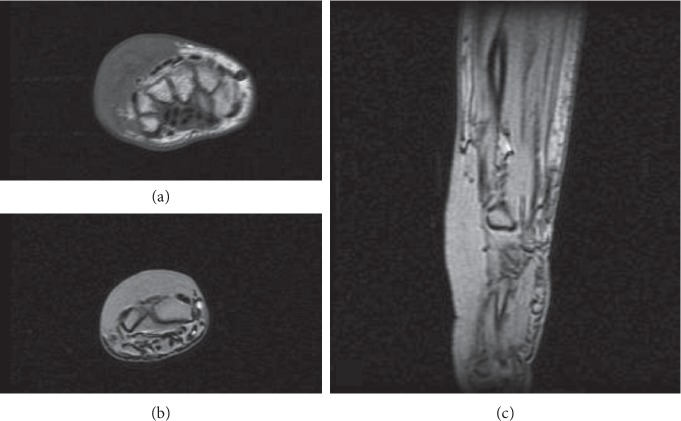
Magnetic resonance images in T1-weighted axial view (a), T2-weighted axial view (b), and T2-weighted sagital view (c), identifying a lesion on the dorsal plane of the right wrist, extending to the inner face but without vascular or tendinous invasion, measuring about 100 × 60 × 18 mm in diameter, with well-defined limits.
